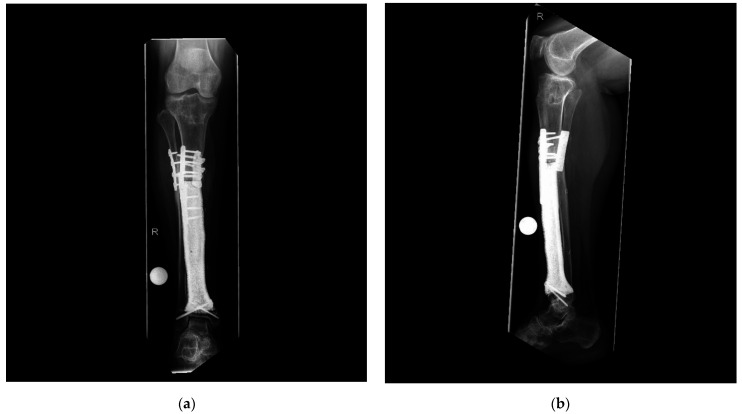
Patient #1: Postoperative radiographs after osteosynthetic plating of the proximal implant-bone interface (a) a.p. view; (b) lateral view. R means right.
